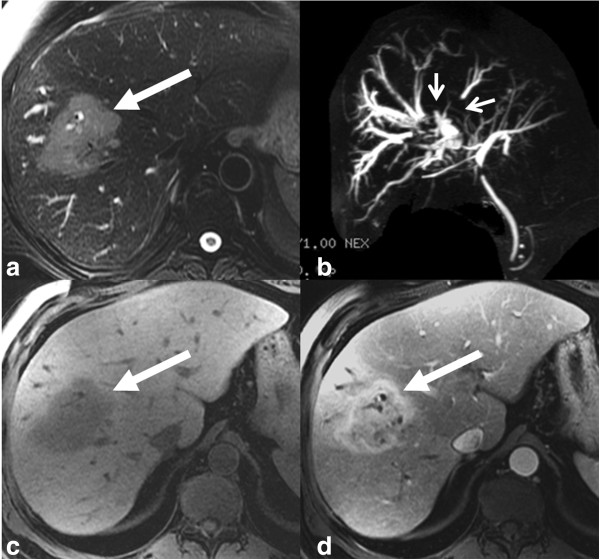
Mixed type iCCA. T2-weighted axial (a), MRCP (b), T1-weighted axial (c) and post contrast T1-weighted axial (d) images demonstrating a predominantly periductal thickening (stricturing iCCA) and also mass forming (arrow) in the right lobe liver. Note the separation of the right intrahepatic ducts on MRCP (arrowheads).
